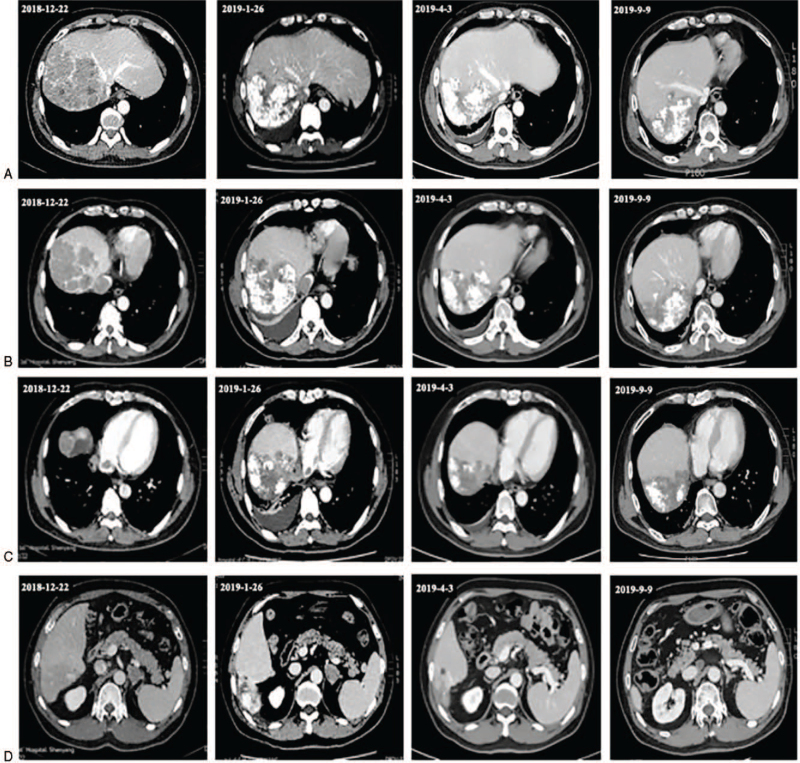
Tumor changes were observed by enhanced CT from December, 2018 to September, 2019. A: Lipiodol deposits are seen in the liver tumor, and the tumor has shrunk after treatment; B: The tumor thrombus of inferior vena cava shrank significantly; C: The right atrial tumor thrombus disappeared in April, 2019; D: Left adrenal gland metastasis disappeared in April 2019. CT = computed tomography.
